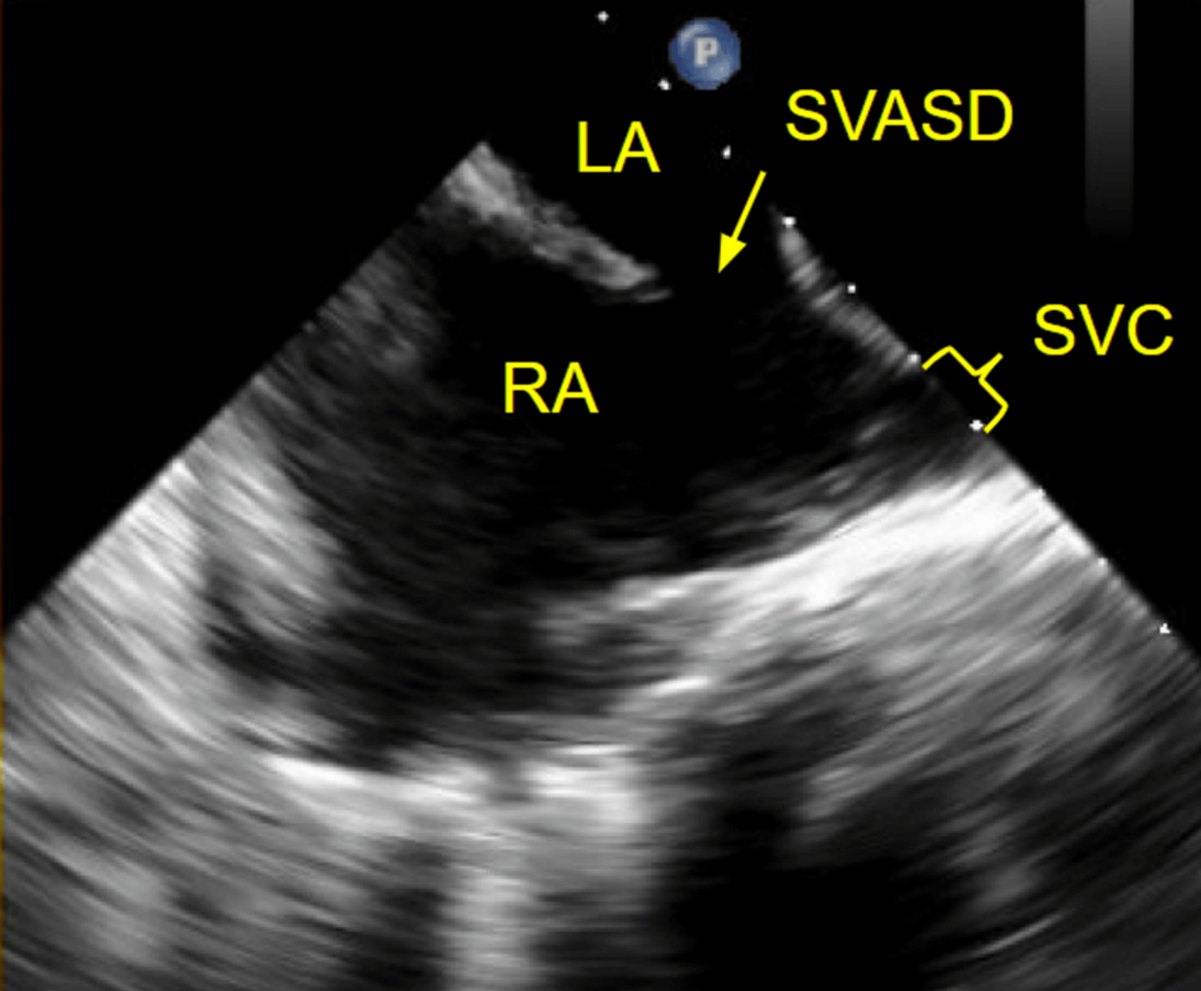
Transesophageal echocardiogram demonstrating sinus venosus atrial septal defect Transesophageal echocardiogram demonstrates sinus venosus atrial septal defect at the posterior aspect of the interatrial septum near the junction of the superior vena cava in conjunction with the right atrium. LA: Left atrium; RA: Right atrium; SVASD: Sinus venosus atrial septal defect; SVC: Superior vena cava.
